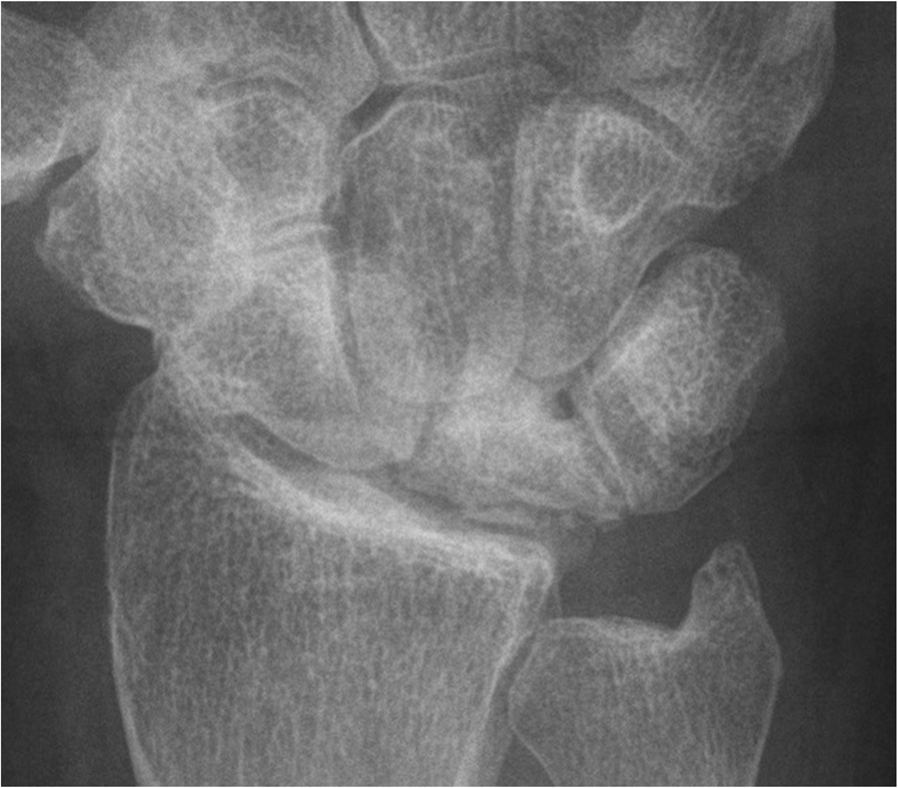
Post-operative x-rays of the right wrist of of a 45-year-old male patient who had undergone STT arthrodesis
